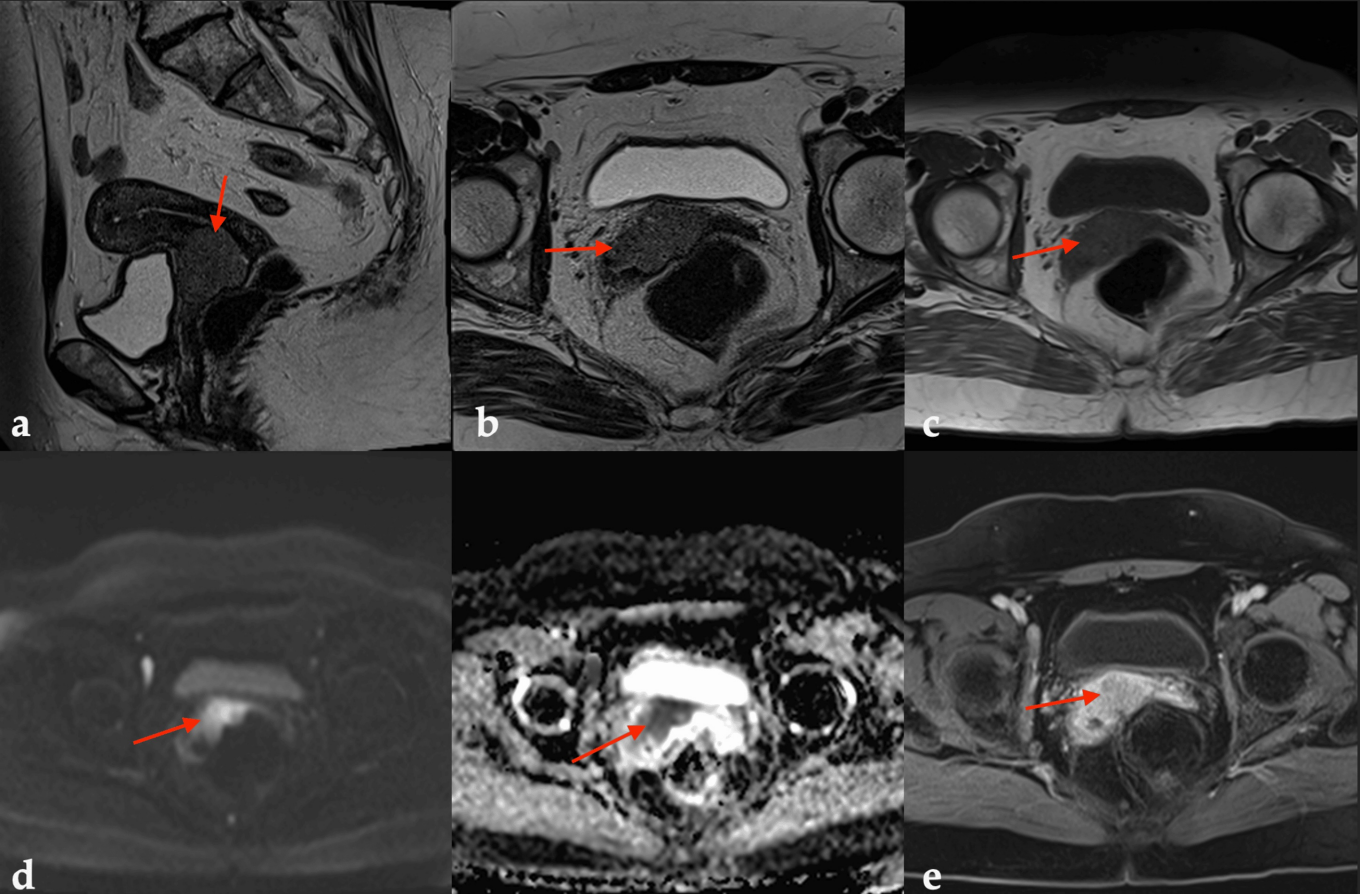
Sixty-four-year-old female patient complaining of post-menopausal bleeding. Pathology revealed invasive non-keratinizing squamous cell carcinoma. (a, b) Sagittal and axial T2WI showed a hyperintense lesion; (c) Axial T1WI revealed an isointense cervical lesion; (d) High signal on DWI and low ADC levels. ADC mean value was (0.63 × 10−3 mm^2^/second); (e) T1 FS postcontrast axial image with heterogeneous enhancement. Red arrows are pointing to the lesion. T2WI: T2 weighted imaging; DWI: diffusion-weighted imaging; ADC: apparent diffusion coefficient; T1 FS: T1 weighted fat-saturated
